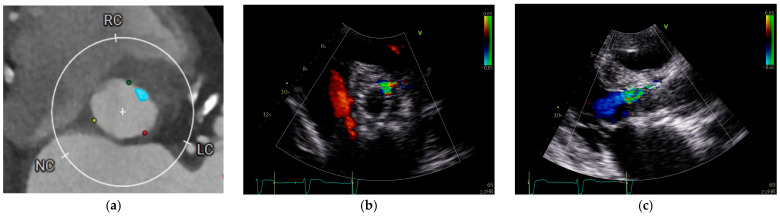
Annular calcification and residual PVL after TAVR. (a) Cardiac computed tomography performed for pre-TAVR planning revealed an annular calcification located at the level of virtual basal ring between the left and right coronary cusps. (b,c) Following TAVR, an anterior paravalvular leak persisted at the site of the previously documented calcification, approximately at the 1 o’clock position.
